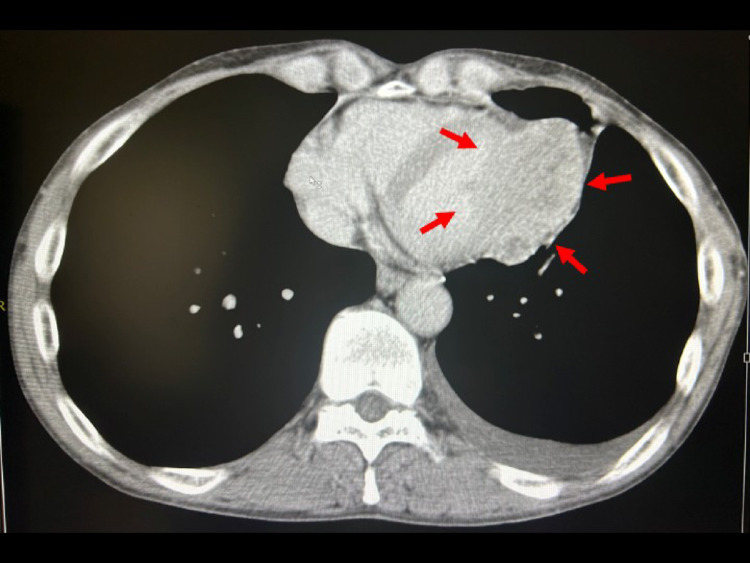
Contrast-enhanced CT of recurrent thymoma with myocardial invasion. Contrast-enhanced computed tomography shows a recurrent thymoma infiltrating the lateral wall of the left ventricle with pericardial involvement. A well-enhancing mass measuring approximately 60 mm is observed
